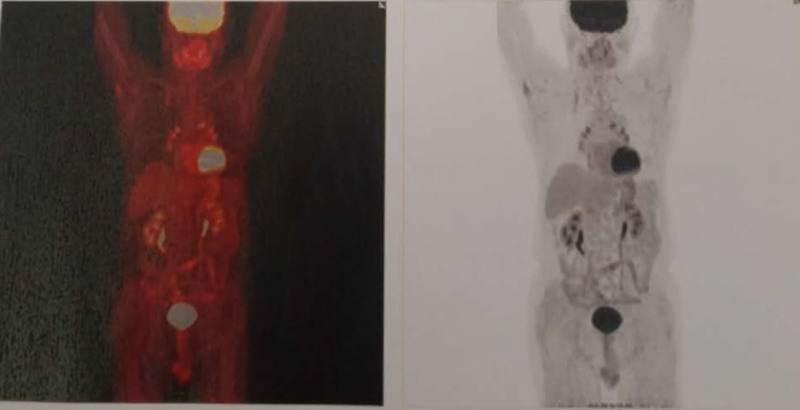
PET/CT (p17102705-2). The mass of hypermetabolic soft tissue in the left hilar region disappeared compared with the 2017.10 PET/CT result; the hypermetabolic enlarged lymph nodes in the original mediastinum and left hilar almost disappeared; there were multiple slightly hypermetabolic lymph nodes in the mediastinum and right hilum, some of which were accompanied with calcium deposition and possibly due to inflammatory proliferation, so regular follow-up checks were required; the left intrapulmonary metastasis disappeared; no abnormal uptake of imaging agents was found in the right 10th rib and left occipital lobe, though regular check was still required.
